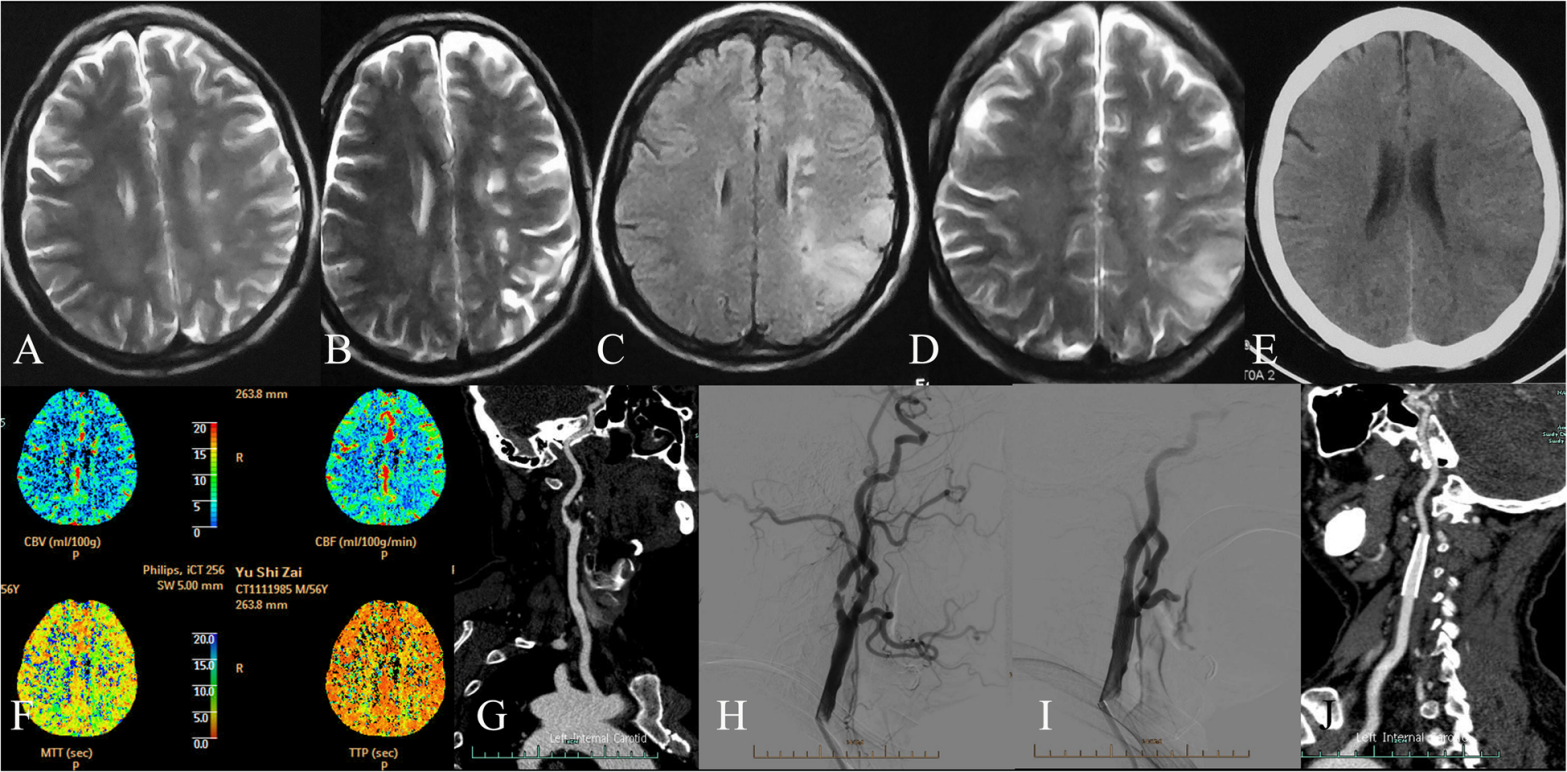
Patient images before carotid artery stenting. a (T2WI [2013-5-5]) and b (T2WI [2013-10-14]):T2-weighted image shows internal border zone cerebral infarction. c, d (2014-12-2): T2 flair image and T2-weighted image showed hyperintensity in the left temporal and occipital lobes. e (2014-12-26): CT image showed low density in the left internal border zone and temporal and occipital lobes. f CT perfusion image showed mild cerebral ischemia of left cerebral hemisphere. g CT angiography (2015-01-03) showed severe left carotid artery stenosis. h, i DSA showed severe left carotid artery stenosis with stenting therapy. (j): 3-month follow-up CT angiography of left carotid artery after carotid artery stenting
